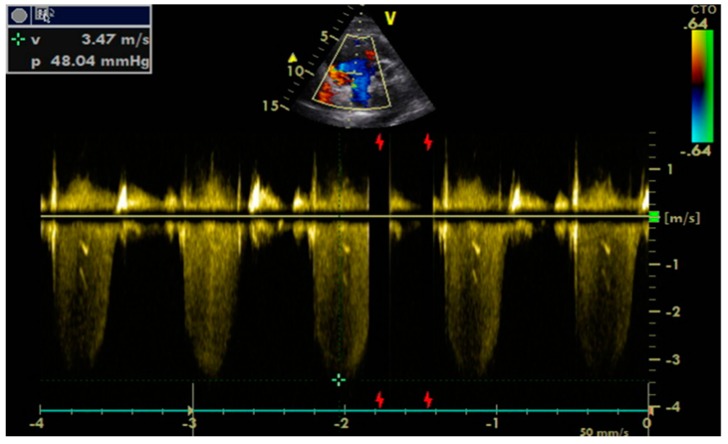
Echocardiographic assessment of tricuspid regurgitation peak velocity (TRpV) by continuous Doppler (CW) on tricuspid regurgitation jet. V: velocity; P: gradient (is 4V^2^). V: specific echocardiographic parameter (it does not mean a measurement data).
